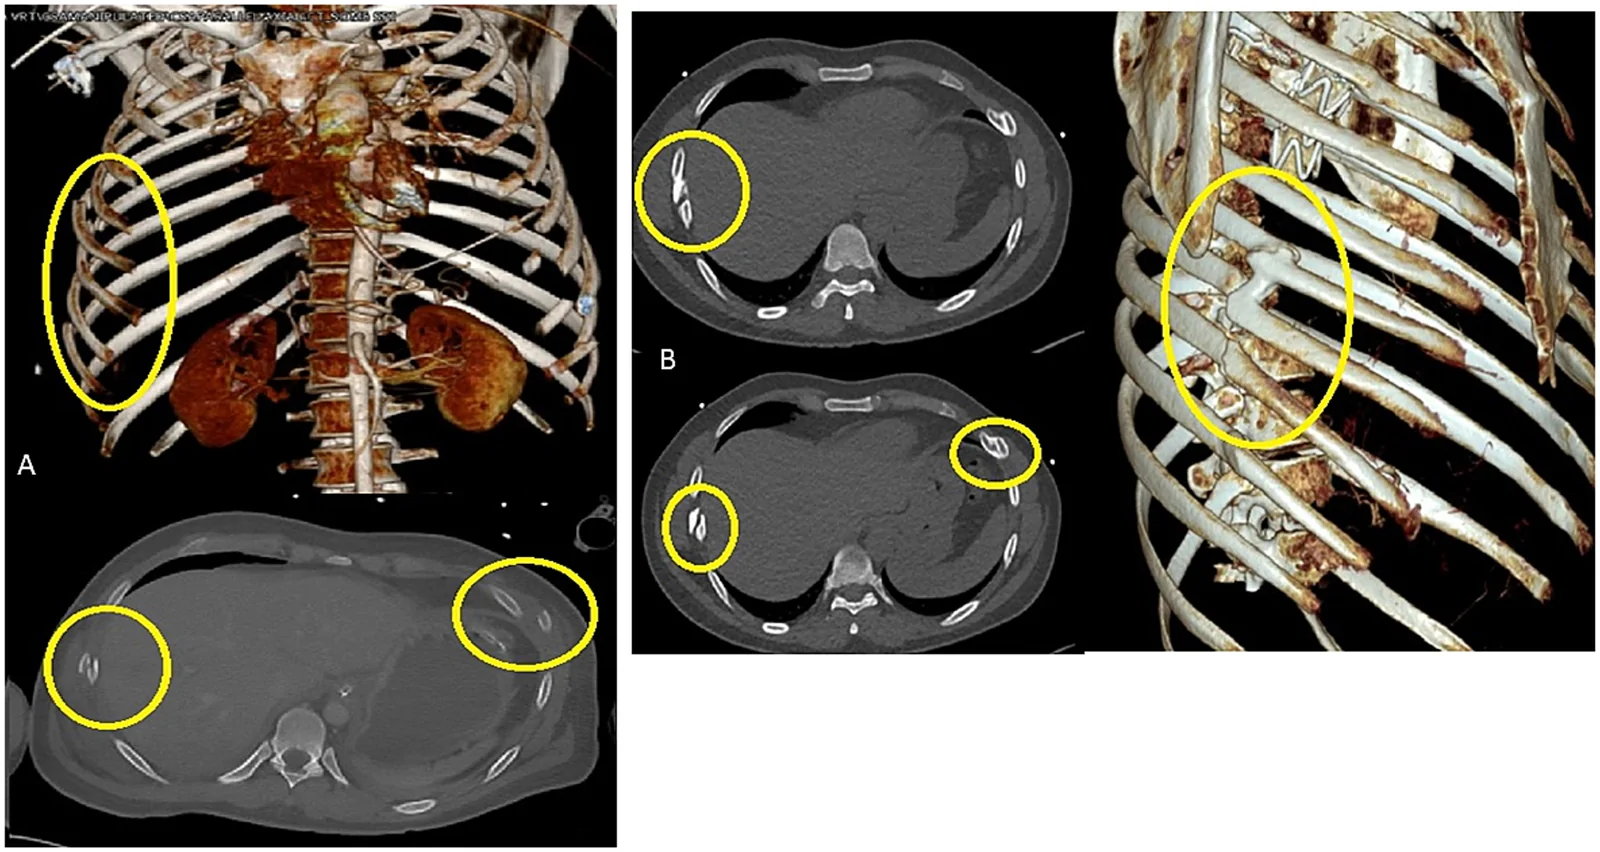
(A) Initial CT with 3D reconstruction showing bilateral fractures; (B) CT with 3D reconstruction showing malunion of the sixth and seventh rib with heterotopic ossification and nonunion of the eighth rib.
